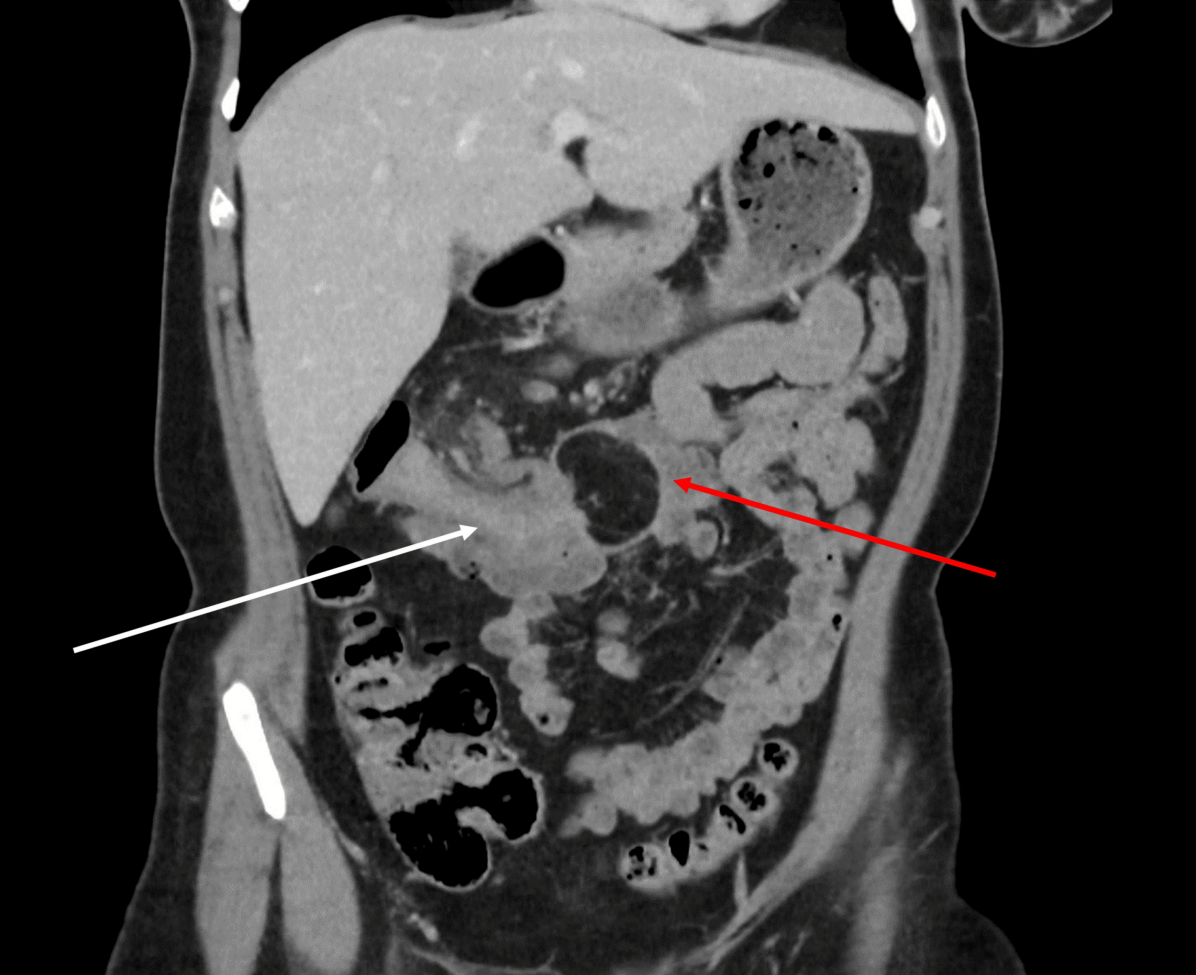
Coronal slice of CT scan in the portal venous phase, with the red arrowing indicating the site of the lipoma, and the white arrow indicating the site of intussusception
